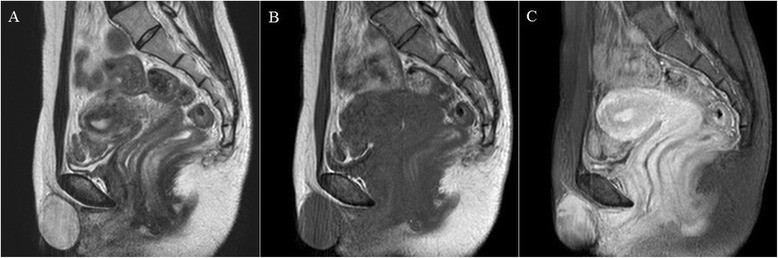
Sagittal T2-weighted (A), T1-weighted (B), and post-contrast T1-weighted (C) magnetic resonance images.
